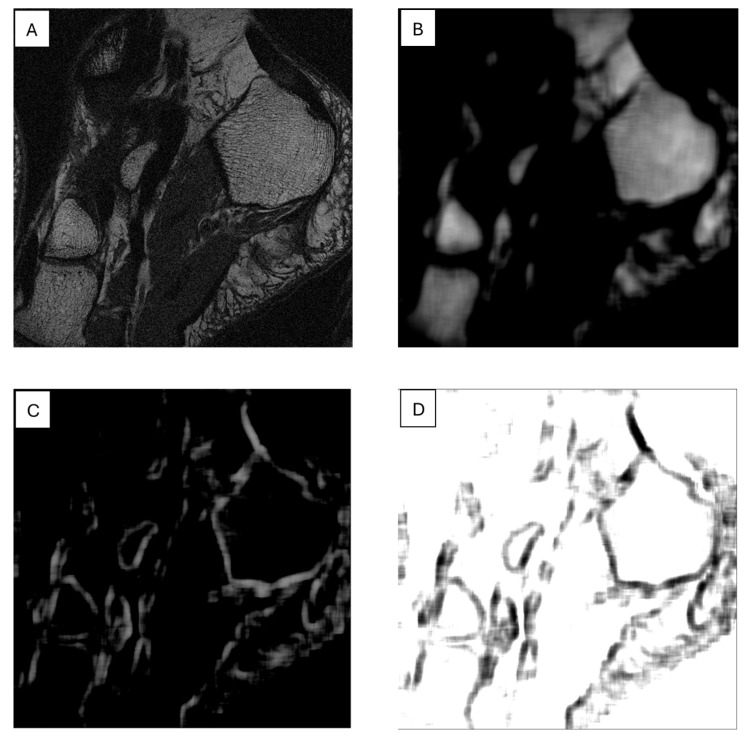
T1W MR image of the foot bone (A); feature maps calculated for this image: SumAverage (B); SumOfSquares (C); and Sigma (D).
